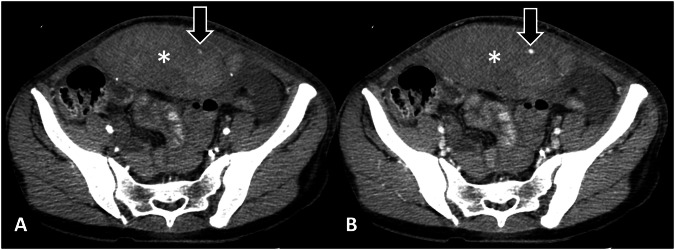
Slow bleeding and its small sizes in the portovenous phase and minimal change between phases. Axial CT images in arterial (A) and portovenous phases (B) of a 40-year-old woman show a large rectus sheath hematoma (asterisks) with a focus of active vascular contrast extravasation (AVCE; arrows). The AVCE is very small in the arterial phase and changes slightly in the portovenous phase, having a change in its perimeter between the two phases of 22%, consistent with a slow bleeder. The patient’s initial blood pressure was 135/72 mmHg with a pulse rate of 60 beats/min. She was treated successfully with transarterial embolization
